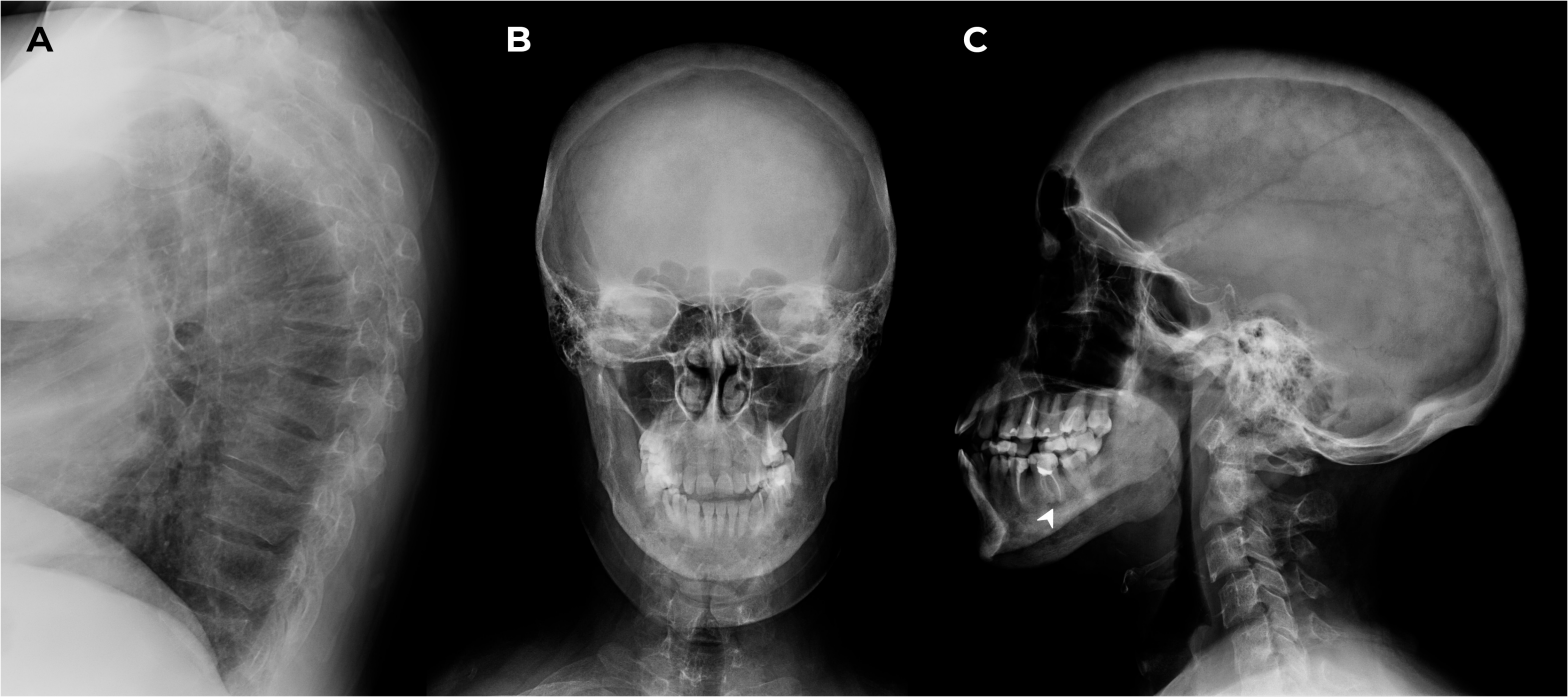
X-ray of the thoracic spine in lateral projection and the skull in frontal and lateral projections. (A) Th_6-8,12_ compression fractures. (B, C) Subcortical resorption on the alveolar masticatory surface (arrow) and multiple bone cysts in maxilla/mandible between 2 and 10.6 mm in the maxilla/mandible, multiple foci of fibrosis and endosellar calcification of the dura mater.
